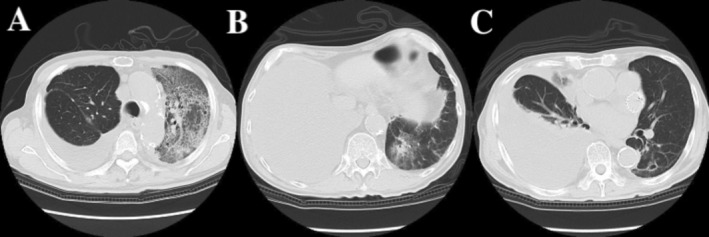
Imaging of adverse events during sequential MET‐TKI therapy. (A) Diffuse ground‐glass opacities in the left lung observed on CT after 2 months of tepotinib therapy, consistent with Grade 3 drug‐induced ILD. (B) Newly developed ground‐glass opacities after 10 days of capmatinib therapy, raising suspicion of ILD (Grade 1). (C) Chest CT on Day 36 of gumarontinib therapy. No ILD findings were observed, although right pleural effusion was present. Gumarontinib was discontinued due to Grade 2 hepatotoxicity.
